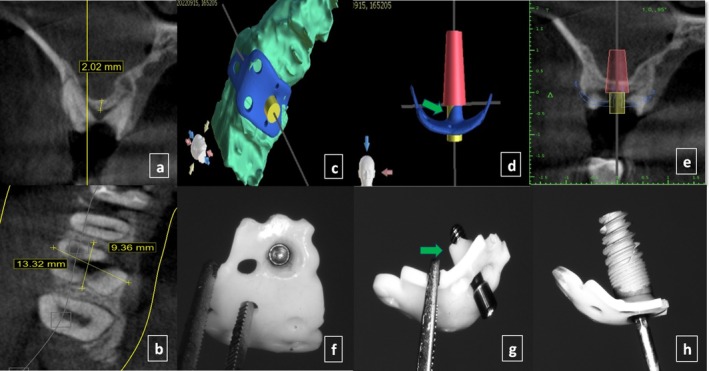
Digital planning of the implant and CZM in the maxilla. (a, b) CBCT measures; (c) ZM in blue and axis of the future abutment in yellow; (d) connection of the implant (red) to the ZM connector at the inner surface (green arrow); (e) position of the assembly ZM/Implant; (f) ZM for clinical use with perforations; (g) the ZM connector; (h) screw fixation of the implant to the ZM.
